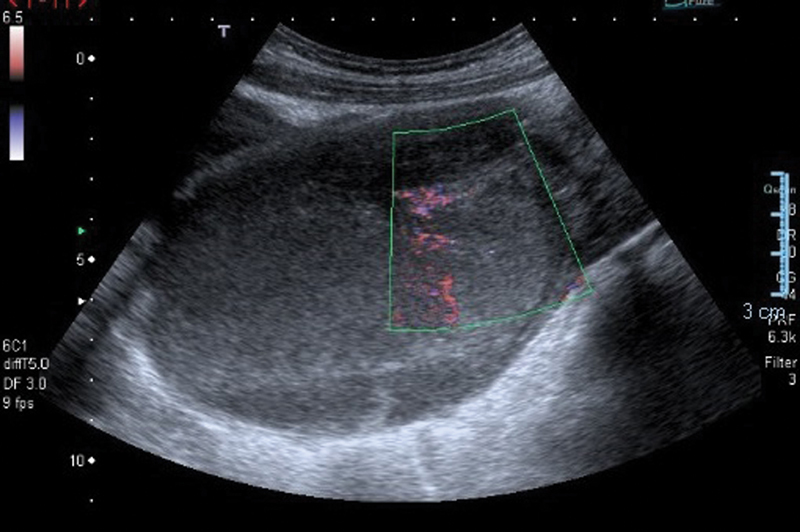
Abdominal ultrasound showing a distended uterus.
